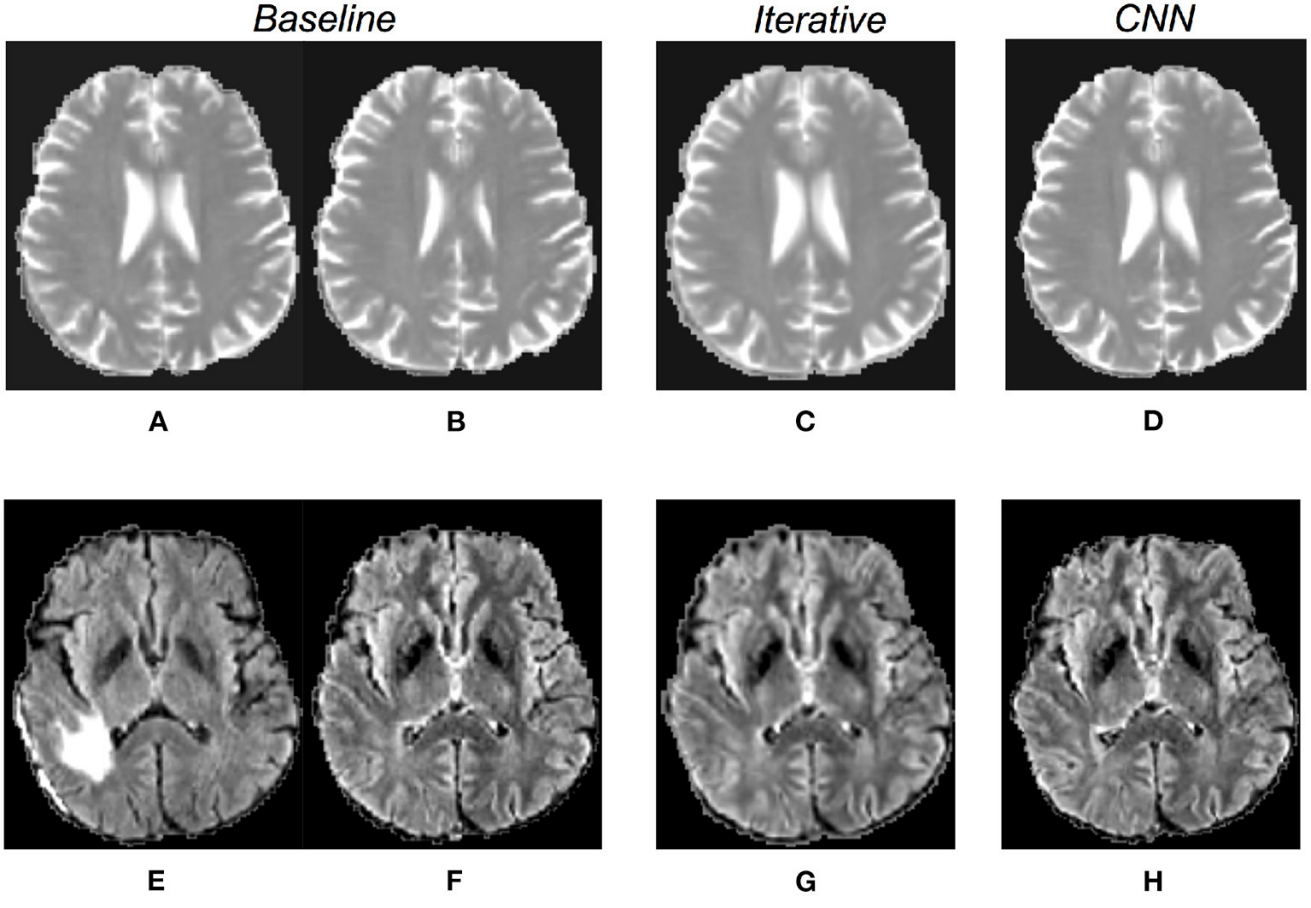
Qualitative results obtained by CNN and iterative solutions masking the distance measure. The first row is related to methods using the T2 MRI sequence, whereas the last one shows example results for solutions using the FLAIR sequence. The first two columns show the corresponding slices of the pre- and post-operative volumes (A,B,E,F), rigidly registered in the pre-processing step. The third column presents warped moving images obtained by the iterative method (C,G), the fourth column shows the results for the CNN models (D,H).
